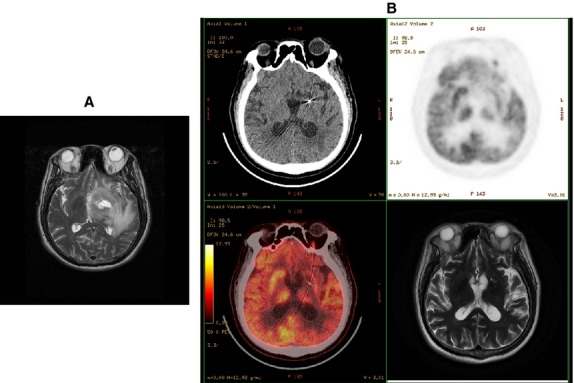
In Patient 1, MR scan shows a mass lesion on the left basal ganglia (A). After stereotactic brachytherapy, chemotherapy and reduced external RT, CT/PET-CT/MR images show the lesion disappeared (B).
